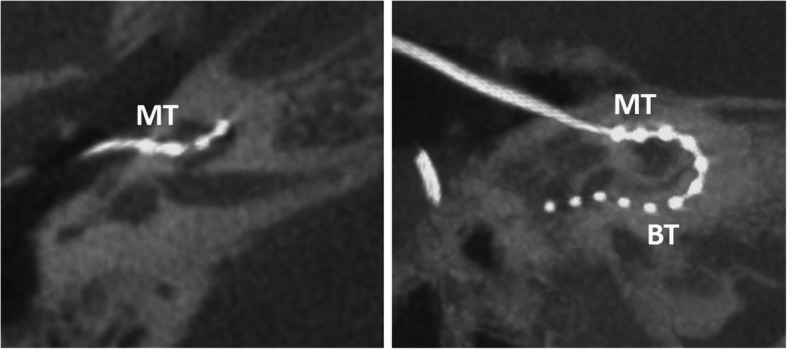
A 51-year-old male patient, with normal postoperative finding after retrograde electrode surgery due to cochlear fibrosis. CBCT axial image (left image) shows electrode entering at the middle turn (MT). CBCT paraaxial maximum intensity projection image (right image) shows the electrode running down the basal turn (BT). The two most distal electrode contacts are in the tympanic space and need to be deactivated
